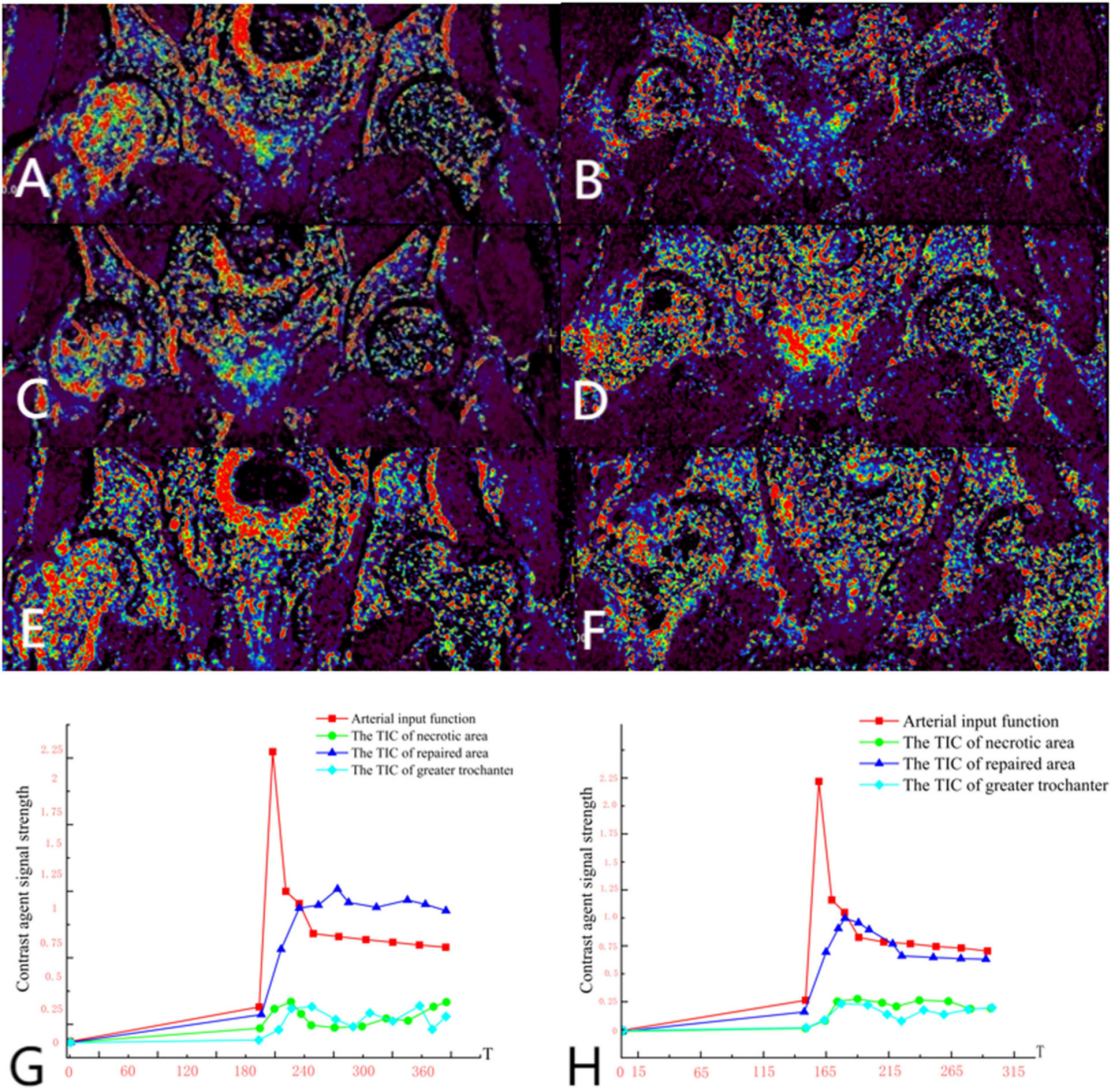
(A,C,E) Preoperative DCE-MRI Ktrans pseudocolor maps; (B,D,F) postoperative. (G) Arterial input function and time-intensity curves (TIC) for necrotic area, repaired area, and greater trochanter preoperatively. (H) Arterial input function and time-intensity curves (TIC) for necrotic area, repaired area, and greater trochanter postoperatively. The perfusion in the anterior and posterior necrotic area increased after operation, while the abnormal hyperperfusion in repaired area decreased.
